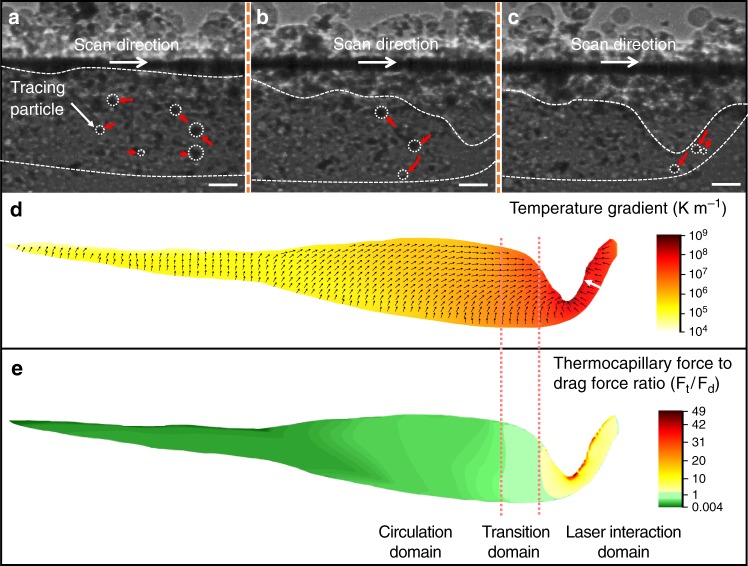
Driving forces for pore motion and elimination. a–c X-ray images showing trajectories (indicated by red arrows) of the tracing particles (tungsten microparticles, marked by white dotted circles), which indicate the melt flow at the circulation domain (a) transition domain (b) and laser interaction domain (c) inside the melt pool during the LPBF process. d Temperature gradient inside the melt pool during LPBF process, obtained by multi-physics modeling with laser processing parameters the same as the in-situ experiments (see “Methods” section). The magnitude and direction of temperature gradient are indicated by color and black arrows, respectively. The white arrow indicates the temperature gradient increases from the solid-liquid interface (melting front) to the depression zone front wall (Fig. 5). e Ratio of thermocapillary force (F_t_) to drag force (F_d_) for a pore with a diameter of 10 µm. In a–c, the laser power is 360 W, the scan speed is 1 m s^−1^ and the thickness of powder layer is 100 μm. Scale bars in a–c are 50 μm
